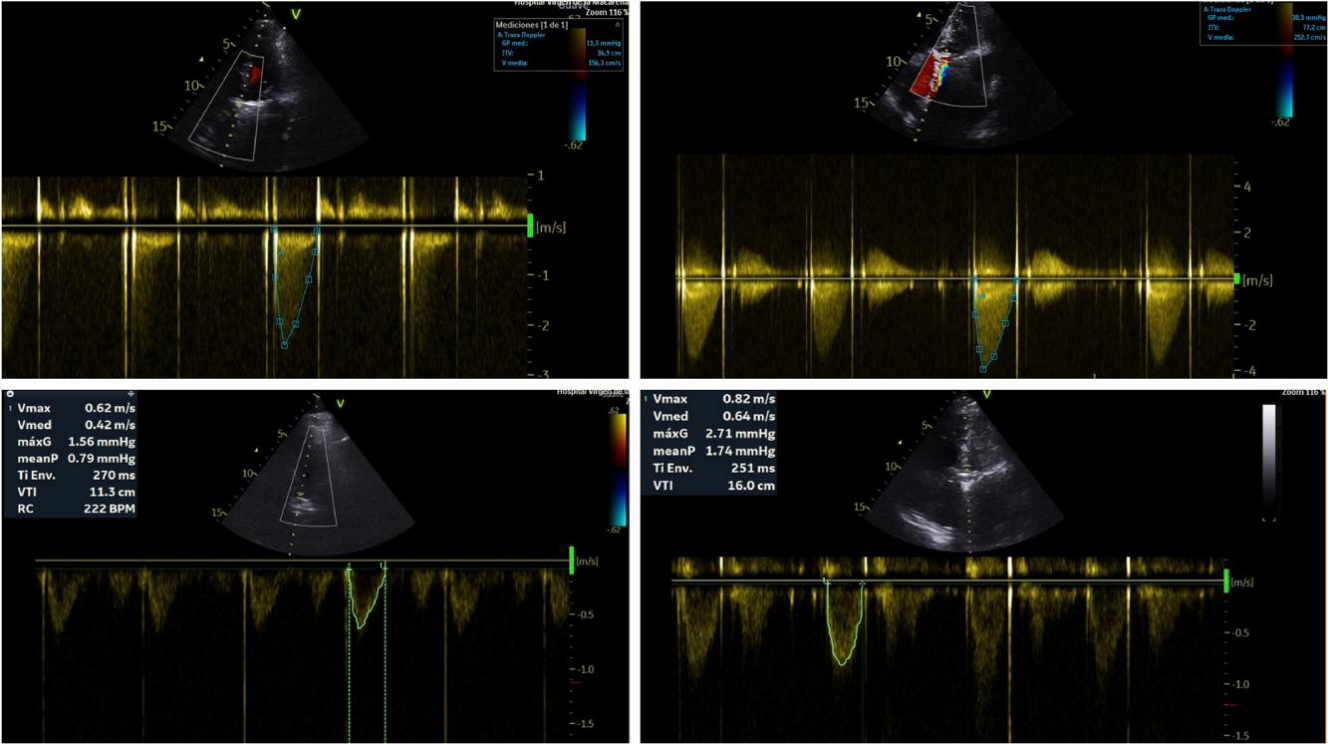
Continuous-wave Doppler. Trend of cardiac output and transaortic gradient.
